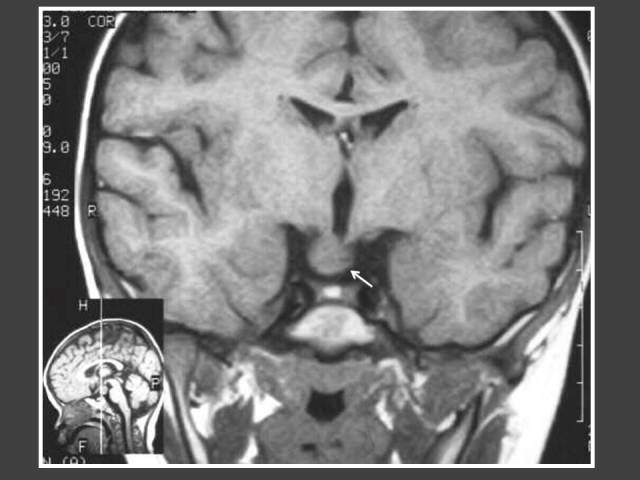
One year-old boy who presented with penile enlargement. Coronal T1WI MRI of the brain shows mass in hypothalamus (arrow) representing tuber cinerium hamartoma.
